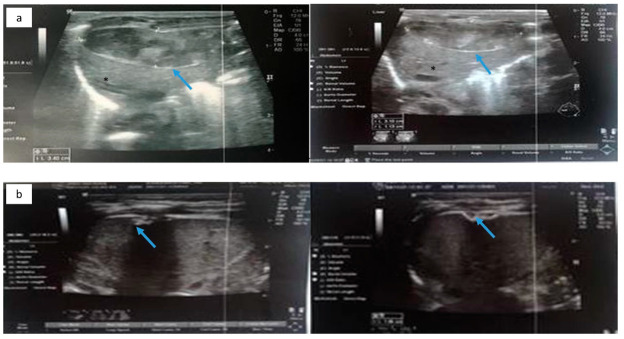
(a) Abdominal ultrasonography depicting hypoechogenic intrahepatic irregular lesion on the right lobe (3.4 cm × 1 cm) (blue arrow); and (b) abdominal ultrasonography depicting the calcific deposits (1 cm × 1 cm) (blue arrow) at the right lobe of the liver. Normal liver parenchyma is noted with asterisk (*).
